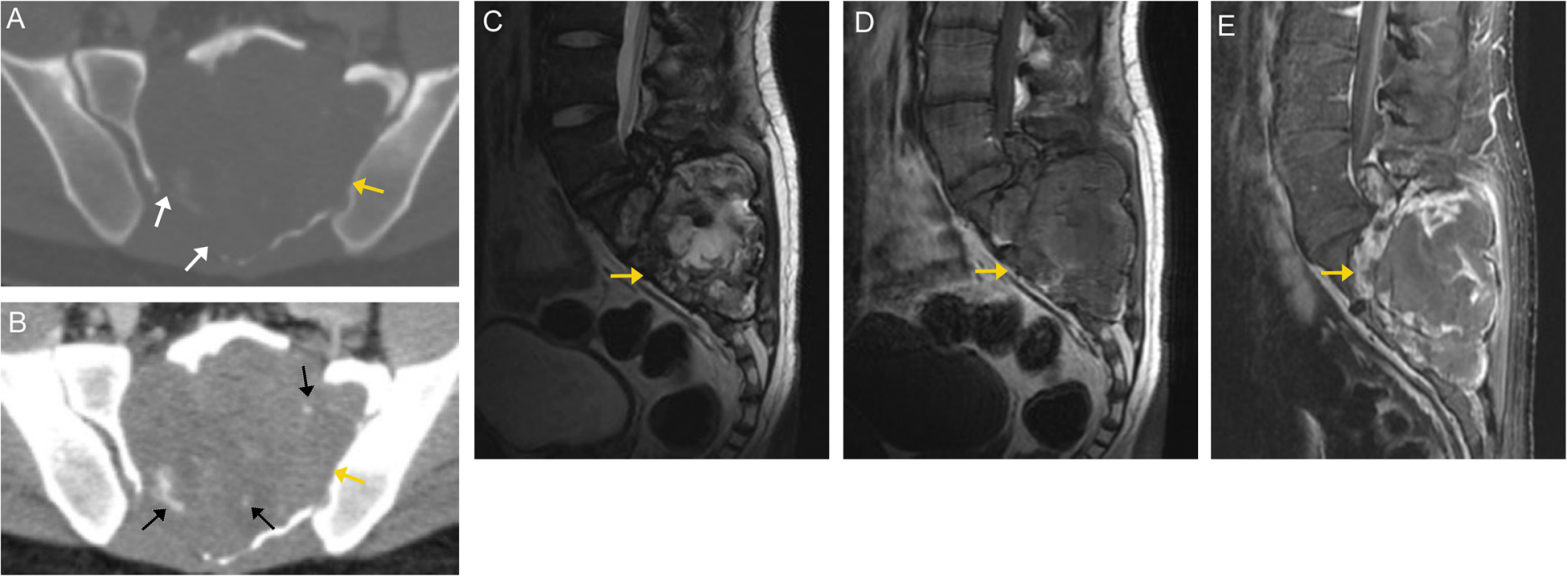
Angiosarcoma. Axial CT image in the bone window (a) demonstrates a large lytic lesion replacing the majority of the sacrum (orange arrow), with multiple areas of cortical erosion and destruction (white arrows). In the contrast-enhanced soft tissue window (b), enhancing areas are present within the mass, representing serpiginous vessels (black arrows). T2 weighted MR image (c) of the lumbosacral spine demonstrates a heterogeneous, destructive mass within the sacrum (orange arrows), with extension into the paraspinal soft tissues. The mass is low to intermediate signal on pre-contrast T1-weighted imaging (d). Post-contrast T1-weighted fat-suppressed image (e) demonstrates heterogeneous enhancement
